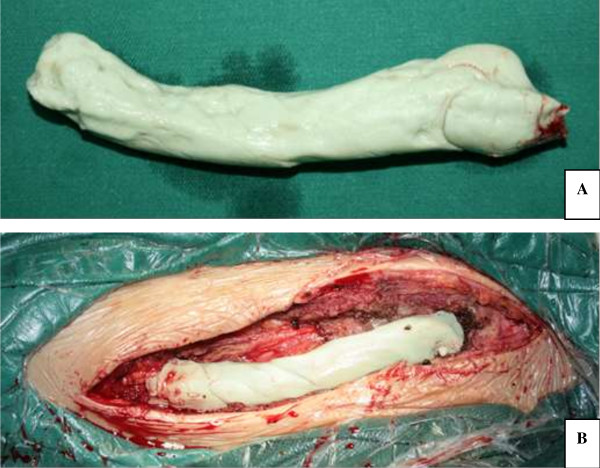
Intraoperative bone cement prosthesis was made by surgeons. A. Size of bone cement prosthesis based on the preoperative CT scan B. Reconstructed bone defect with bone cement prosthesis.
